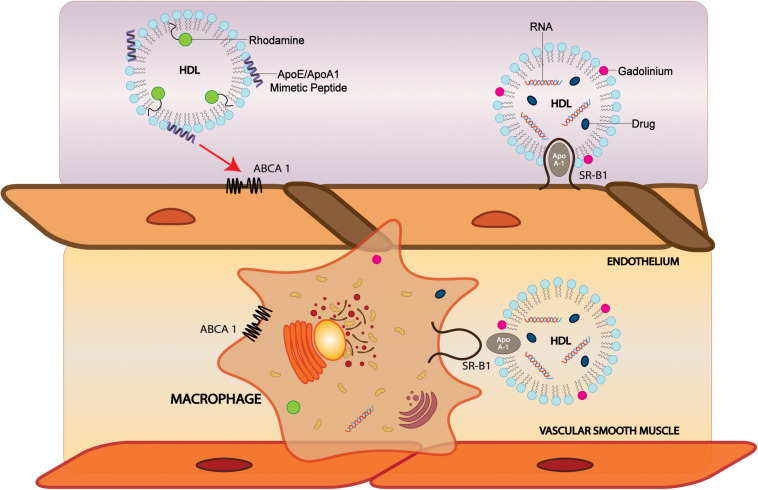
Modification of sHDL particles can facilitate brain, vascular cell, and macrophage delivery. HDL-like NPs labeled with phospholipid-containing dyes such as rhodamine and probes conjugated with CS-6253, an ABCA1 agonist, can target ABCA1 on endothelial cells and macrophages to deliver cargo. Incorporation of MRI contrast agents such as gadolinium into HDL can increase signal. In SR-BI positive cells, sHDLs comprised of apoA-I can bind SR-BI and are taken up by the cell where cargo is delivered. ABCA1, ATP Binding Cassette Subfamily A Member 1; SR-BI, Scavenger receptor, class B type 1.
